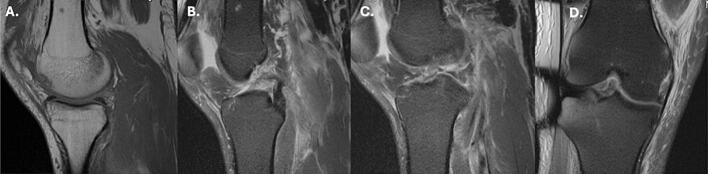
MRI of the right knee demonstrating T1 weighted sagittal view of an osteochondral fracture along the anterior portion of the medial condyle (A), T2 weighted fat-suppressed sagittal views of the complete avulsion of the PCL from its femoral attachment (B) and complete disruption of the ACL (C), and T2 weighted fat-suppressed coronal view of the complete ACL tear (D).
